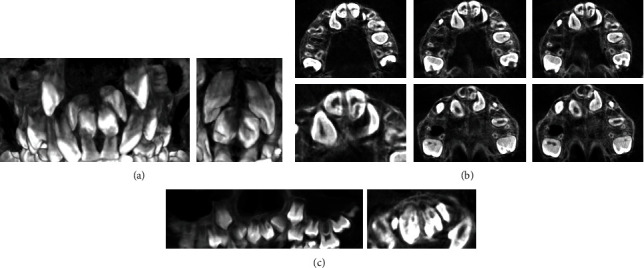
CBCT examination: (a) vestibular and palatal views, (b) transverse sections, and (с) frontal sections.
